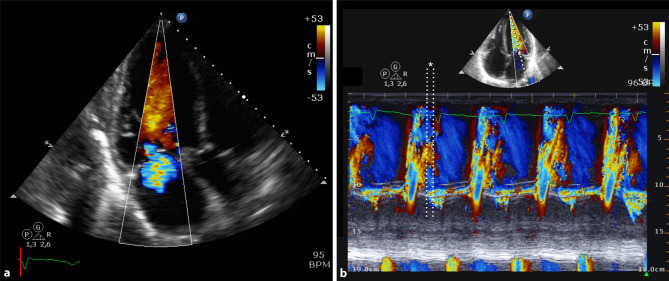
a Colour flow Doppler still frame from apical three-chamber view with diastolic MR; b Colour M‑mode of transmitral flow from the apical four-chamber view with diastolic MR (* indicates the Q‑Mc time). *MR* mitral regurgitation
